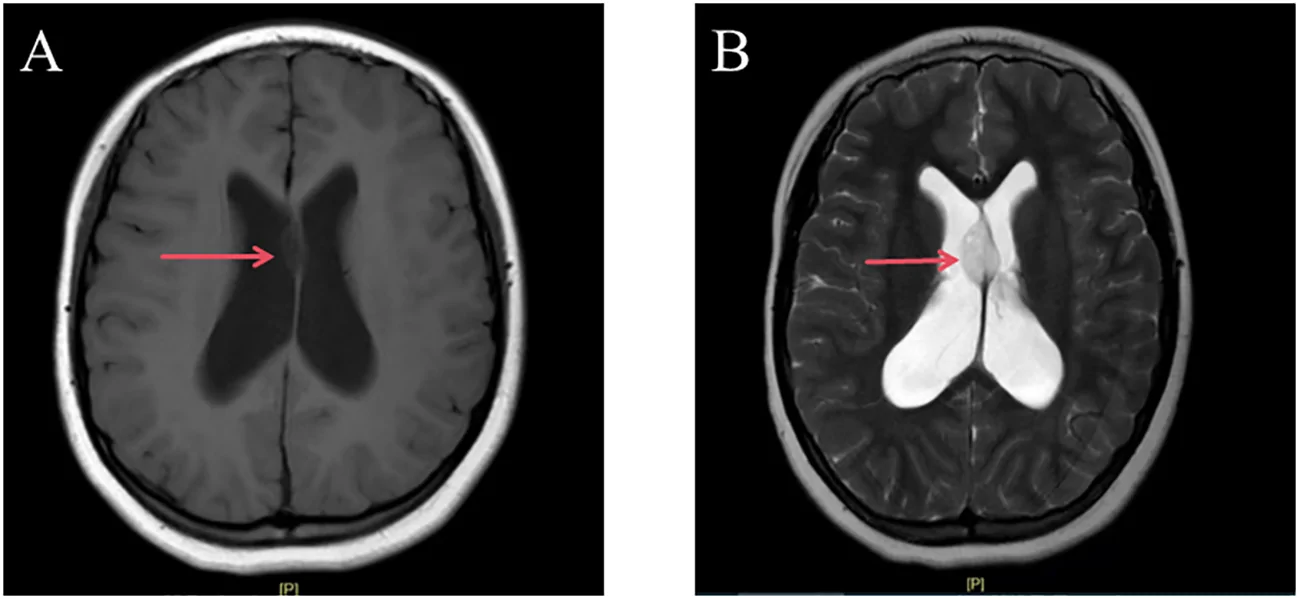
MRI showing bilateral hydrocephalus and a mass at the foramen of Monro. (A) Axial T1-weighted image demonstrating dilated lateral ventricles and a hypointense mass (arrow) at the foramen of Monro. (B) Axial T2-weighted image showing the same mass (arrow) appearing hyperintense, with associated ventriculomegaly.
